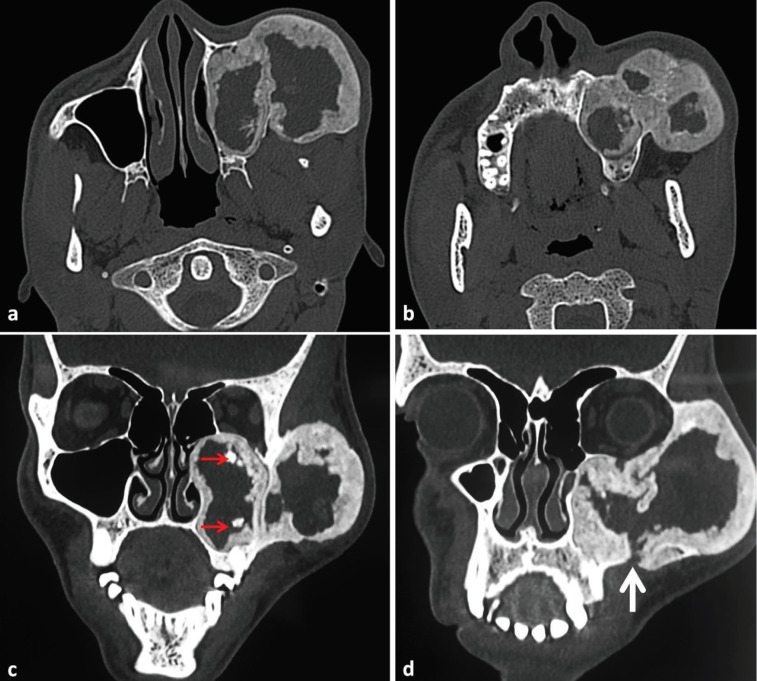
Axial (a, b) and coronal (c, d) sections of computed tomography scan showing well-defined multilobulated expansile lesion involving the left zygoma, lateral orbital rim, infra-orbital rim, zygomatic arch, maxillary sinus and superior alveolar ridge. Foci of ossification noted within the lesion (red arrow) along with area of breach of bone at zygomatic buttress area (white arrow) suggestive of previous site of biopsy
